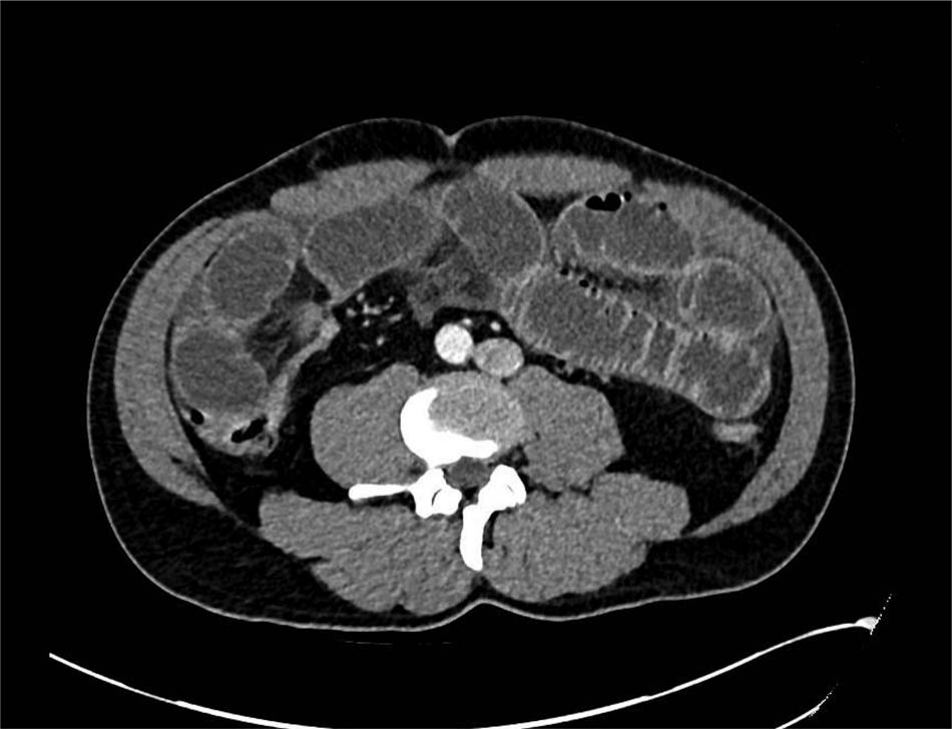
CT showing transition point in right iliac fossa.
